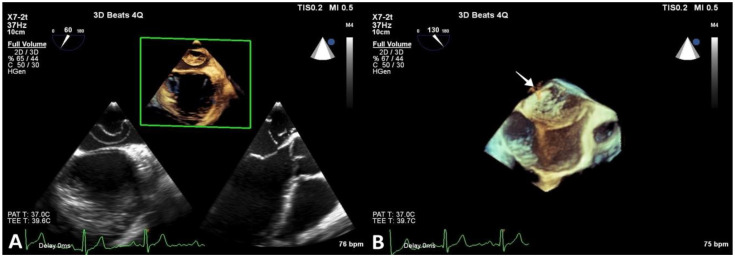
Transesophageal echocardiography revealing CTS. (A) and (B) show 2D and 3D reconstructions visualizing the membrane and its fenestrations (arrow).
